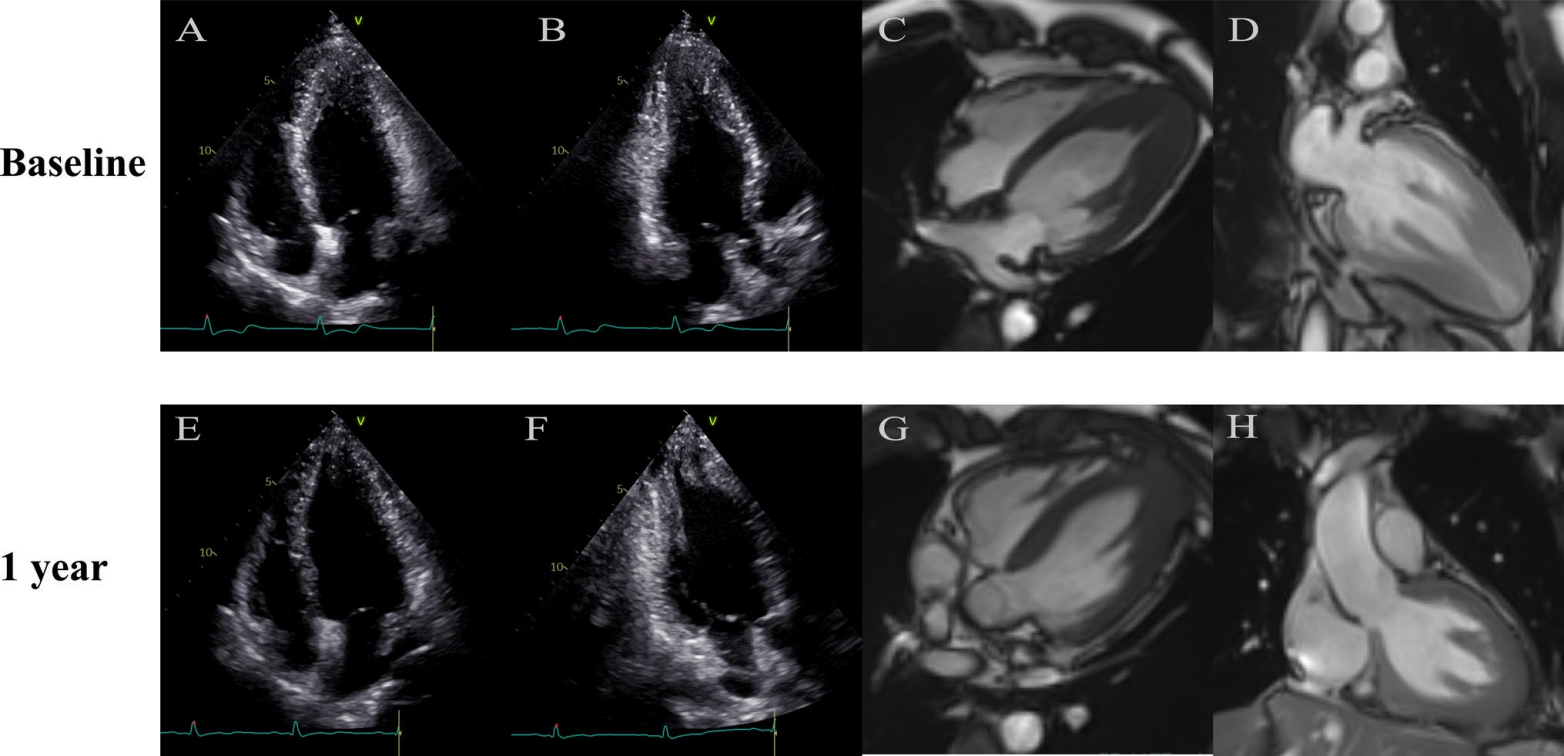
Transthoracic echocardiography (A, four-chamber; B, two-chamber) and cardiac magnetic resonance (C, four-chamber; D, two-chamber) in a patient with ApHCM at baseline. Note the increased thickness of the apex and small end-diastolic volume of the LV. Transthoracic echocardiography (E, four-chamber; F, two-chamber) and cardiac magnetic resonance (G, four-chamber; H, two-chamber) in the same patient one year after TA-BSM. Note the significantly reduced thickness of the apex and the increased end-diastolic volume of the LV. *ApHCM* apical hypertrophic cardiomyopathy, *LV* left ventricle, *TA-BSM* transapical beating-heart septal myectomy
